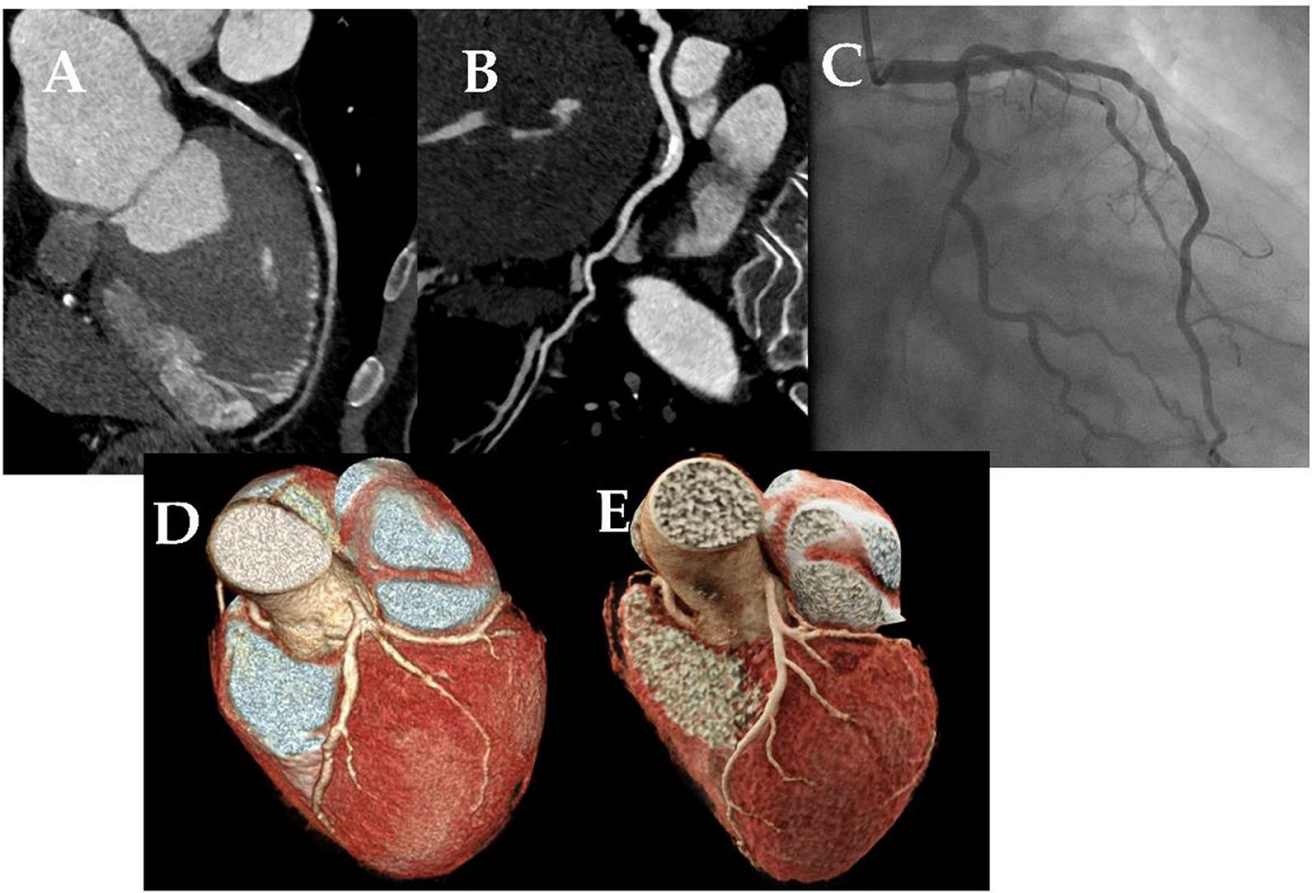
Coronary CTA images from single- source CT scanner (A, D), and dual-source CT scanner (B, E), and diagnostic invasive coronary angiography image (C)
